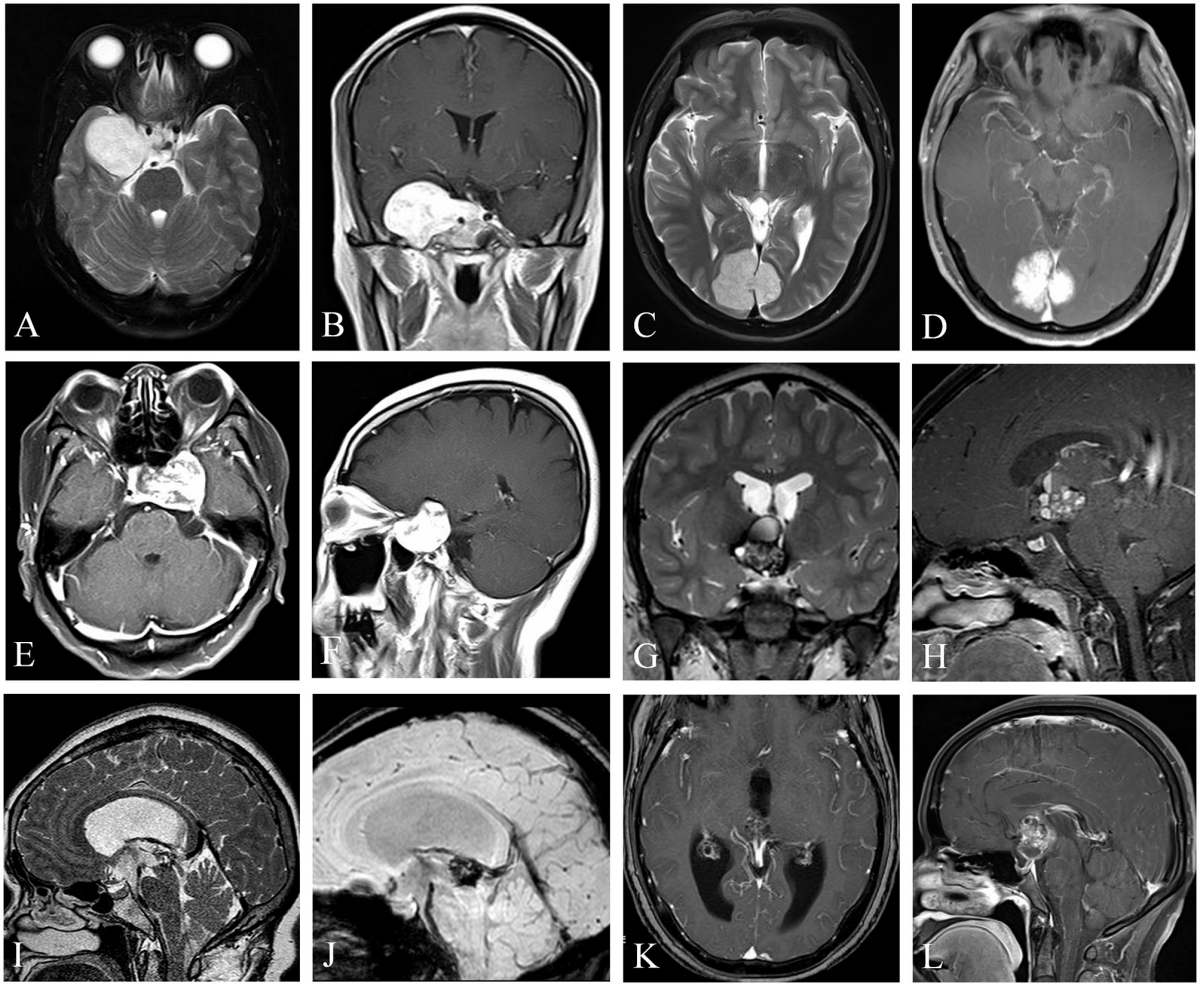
Uncommon cavernous hemangiomas (UCHs) with different locations and radiological features. Case 3, a right enhanced parasellar region CH with pituitary fossa involvement and dural tail sign (A, B), mimicking a sphenoid ridge meningioma. Case 12, a strongly enhanced CH originating from occipital falx was depicted with a dural tail sign (C, D), masquerading as a meningioma. Case 4, a CH located at the sellar or parasellar region, mimicking a meningioma (E, F). Case 10, a CH located in the third ventricle, with classic popcorn-like radiological features, with a hypointense rim on T2-weighted image (G) and heterogenous enhancement (H). Case 11, a CH located at the inlet of midbrain aqueduct (I–K), with susceptibility-weighted imaging (J) showing hemosiderosis deposition of the lesion, indicating a diagnosis of a CH. Case 6, a CH located at the suprasellar region mimicking a craniopharyngioma (L). (I–K) Demonstrated in Neurosurgical Focus Video—*Anterior endoscopic transcortical approach to a pineal region cavernous hemangioma*.
